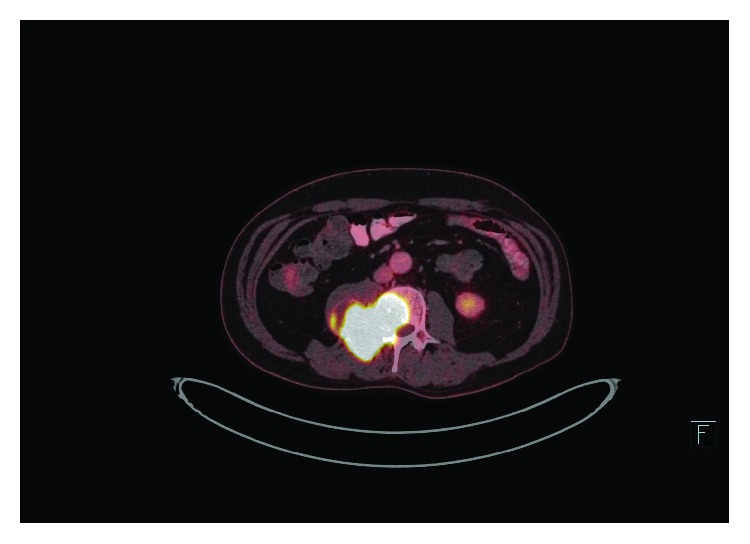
Fused FDG/PET-CT scanning at level of L3/L4 7 months after diagnosis of GBM showing substantial metabolically active metastases in and around L3 and surrounding paravertebral muscle.
